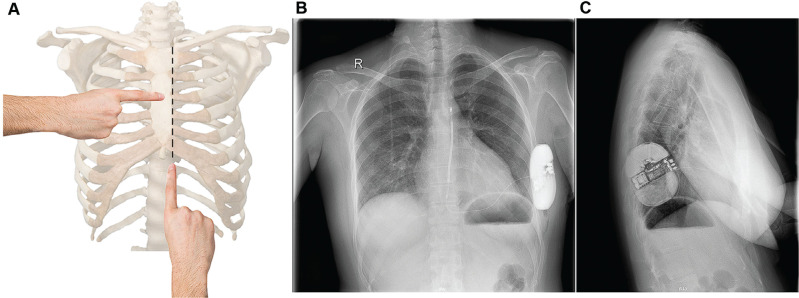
Totally fluoroless subcutaneous implantable cardioverter-defibrillator Implantation (S-ICD). A: Landmarks for totally fluoroless S-ICD Implantation. B: Post-implantation chest X-ray in a 26 year-old female patient with dilated cardiomyopathy.
